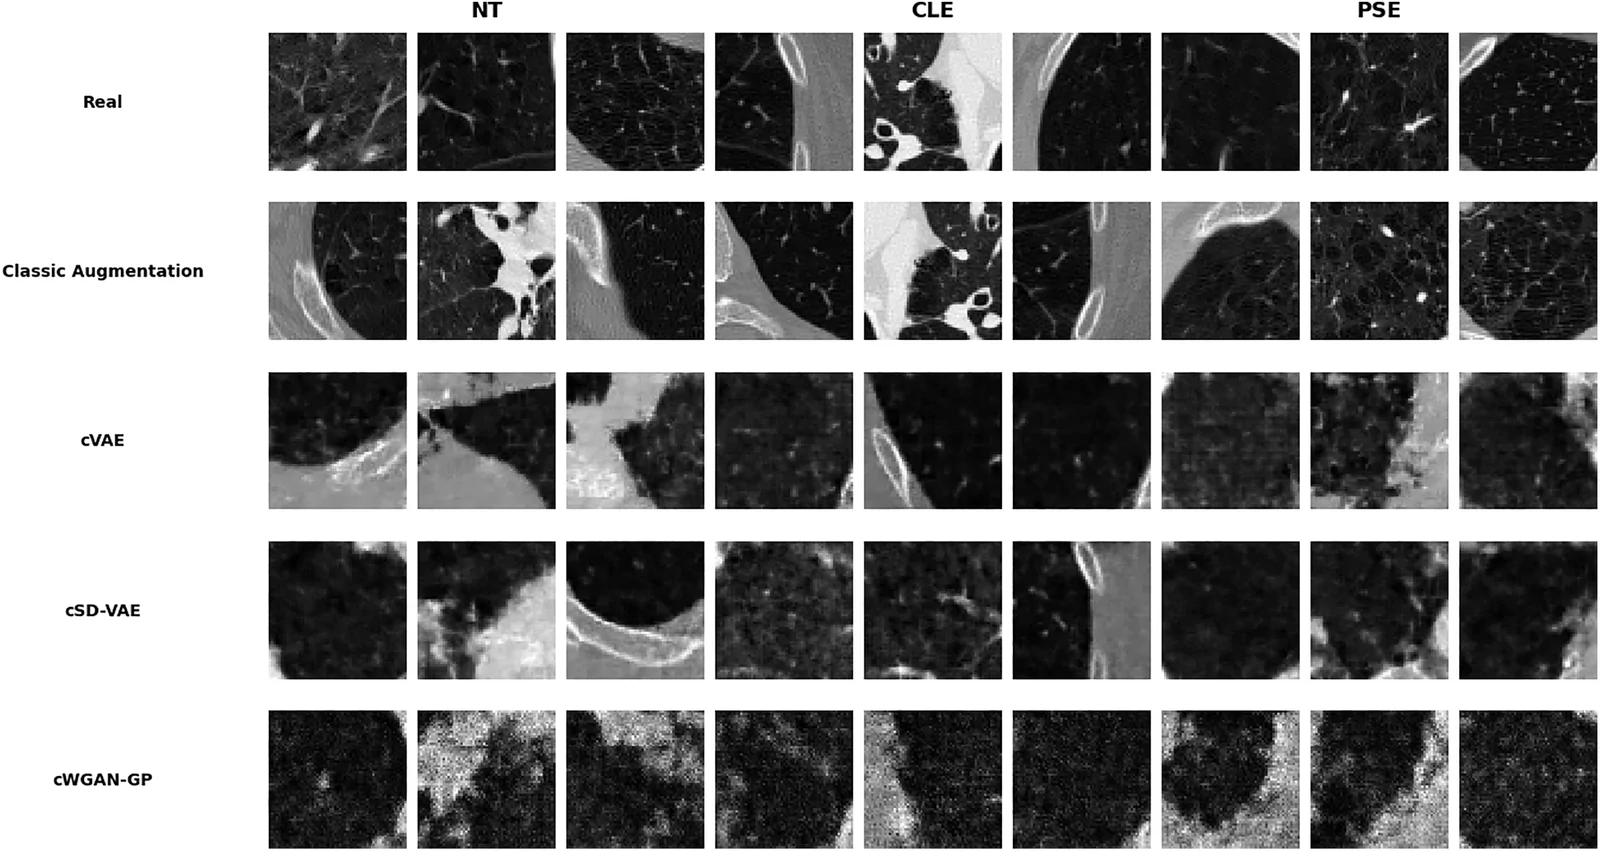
Representative computed tomography (CT) patches by emphysema subtype and augmentation method. Columns correspond to normal tissue (NT), centrilobular emphysema (CLE), and paraseptal emphysema (PSE). Rows show real images, classical augmentation, conditional variational autoencoder (cVAE), conditional shallow-decoder variational autoencoder (cSD-VAE), and conditional Wasserstein generative adversarial network with gradient penalty (cWGAN-GP).
